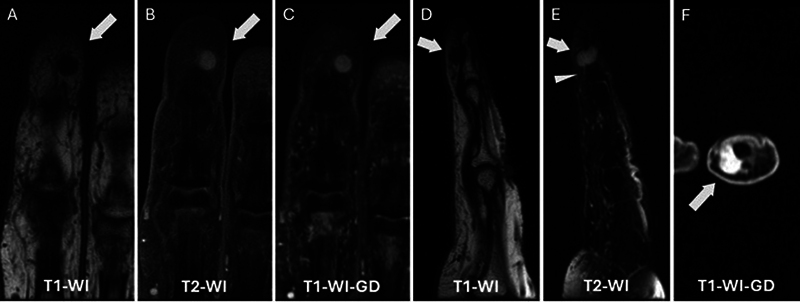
A 60-year-old woman with glomus tumor at the tip of the long finger. Magnetic resonance imaging of the long finger demonstrates a soft tissue nodule at the fingertip, extending from the subungual region to the volar aspect of the distal phalanx, with intermediate signal intensity on T1-weighted images (
